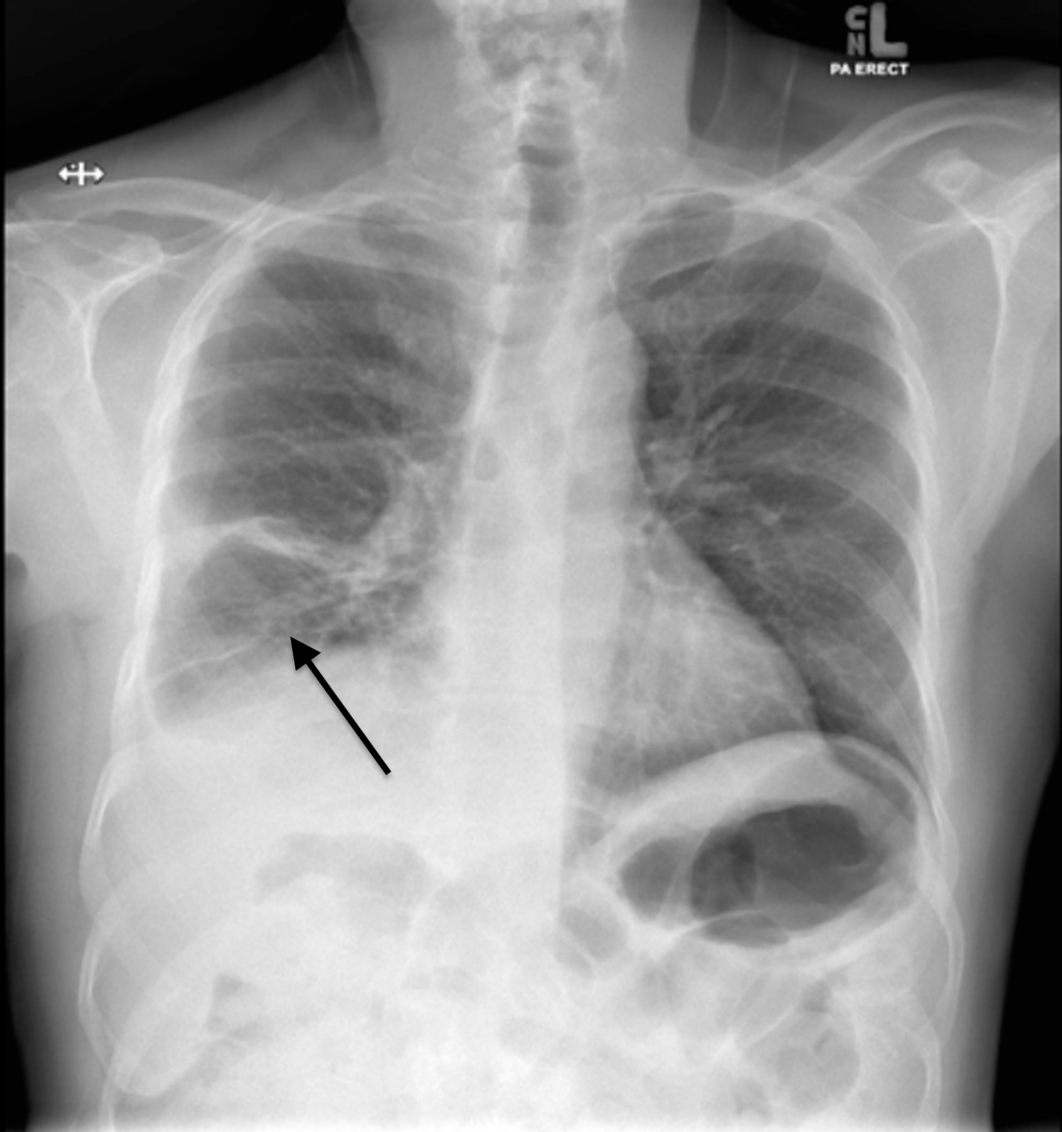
Chest X-ray on follow-up after foreign body removal
